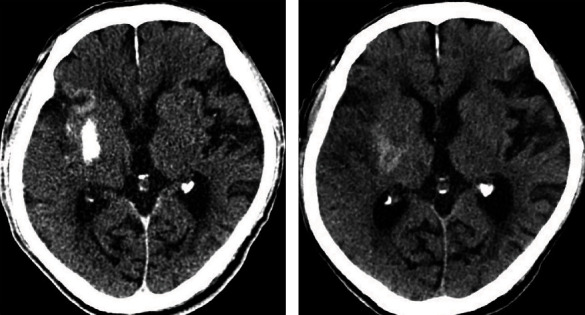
CT scan of a 58-year-old woman who had a sudden onset of impaired left limb movement. (a). The non-enhanced brain CT scan obtained immediately after intra-arterial thrombolysis showing a type II hyperdense lesion in the right lentiform nucleus with a CT value of 90-135 Hu. A type I hyperdense lesion was located in the right insular cortex with a CT value of 52-57 Hu (the latter was not included in this study). There was no mass effect in the above two lesions. (b). Follow-up CT scan obtained 24 hours after the end of AR, the hyperdense lesion of the right lentiform nucleus was obviously absorbed. The patient was not accompanied by deterioration of neurological symptoms.
